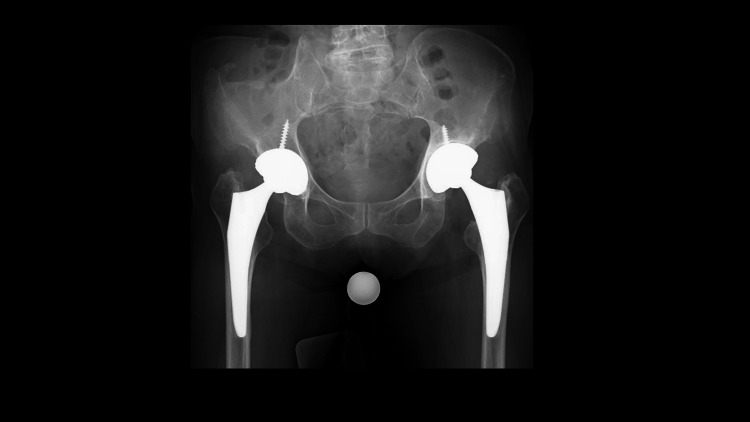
Preoperative hip radiograph A radiolucent line appears in the left acetabular implant and the shoulder of the femoral implant.
